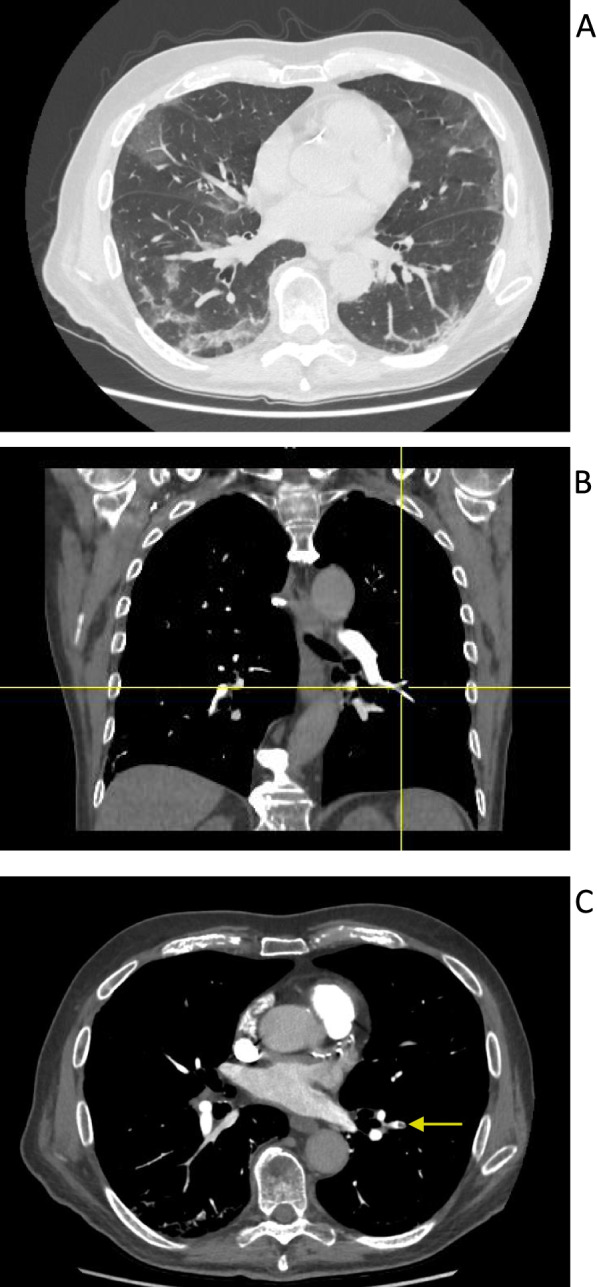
thorax contrast-enhanced CT scan images at admission. A Bilateral ground glass opacities, indicative of interstitial pneumonia. B and C Arterial phase scans indicating a filling defect of the left anteromedial segmental basal artery (pointer in B. and arrow in C)
